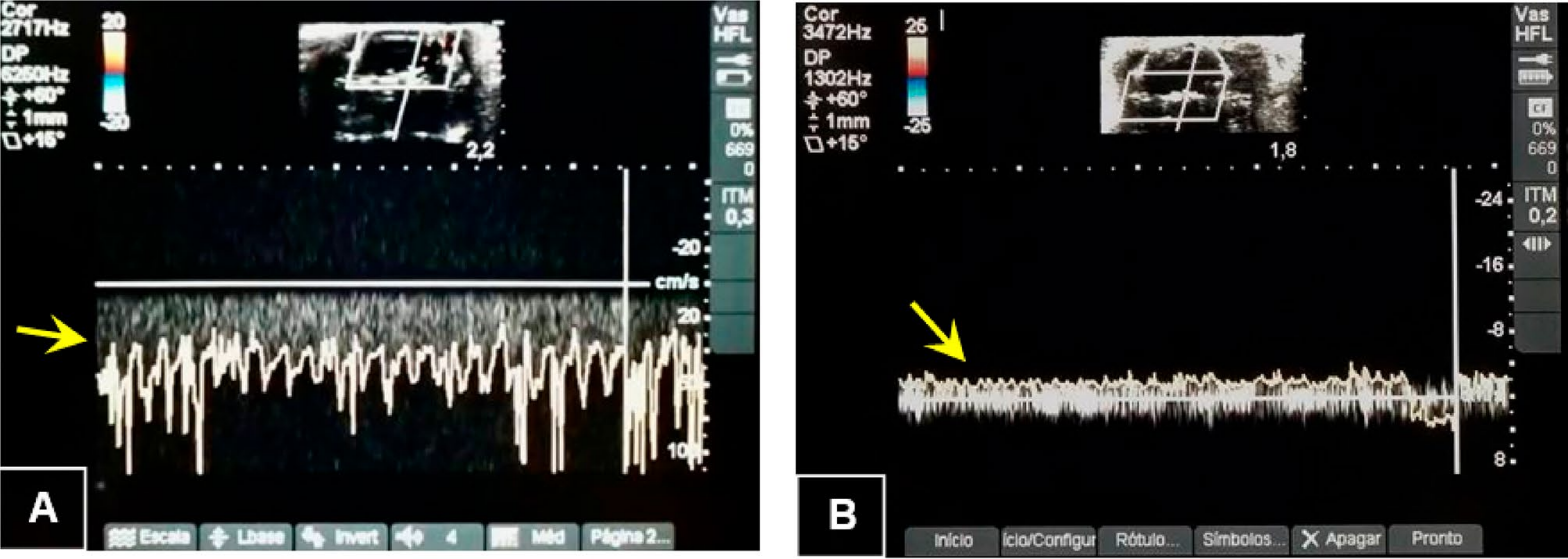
Analysis of cerebral blood flow identified by transcranial color ultrasound. (A) Presence of blood flow in the reverse direction (yellow arrow) before brain death. (B) Presence of flow compatible with brain circulatory collapse (yellow arrow).
